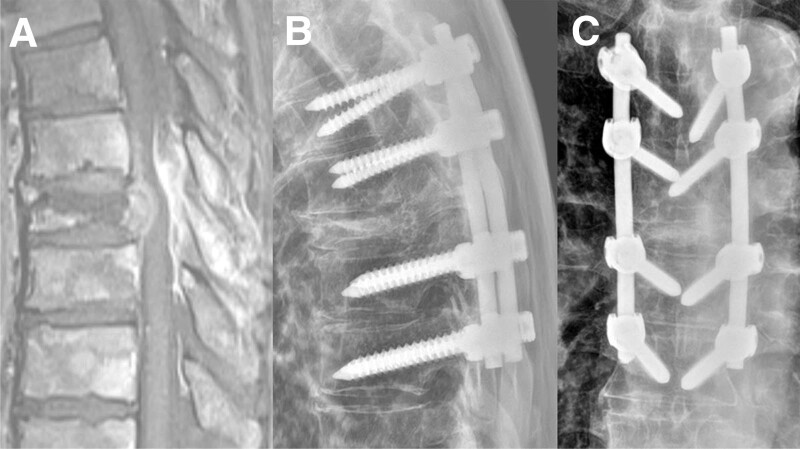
A 65-year-old man diagnosed with prostate cancer presented to the clinic with gait disturbance and back pain. Preoperative sagittal MRI (A) reveals T8 pathologic compression fracture and cord compression. TS is performed, and postoperative lateral (B) and AP (C) X-ray films show the posterior screw fixation from T6 to T10. AP = anteroposterior, MRI = magnetic resonance image.
